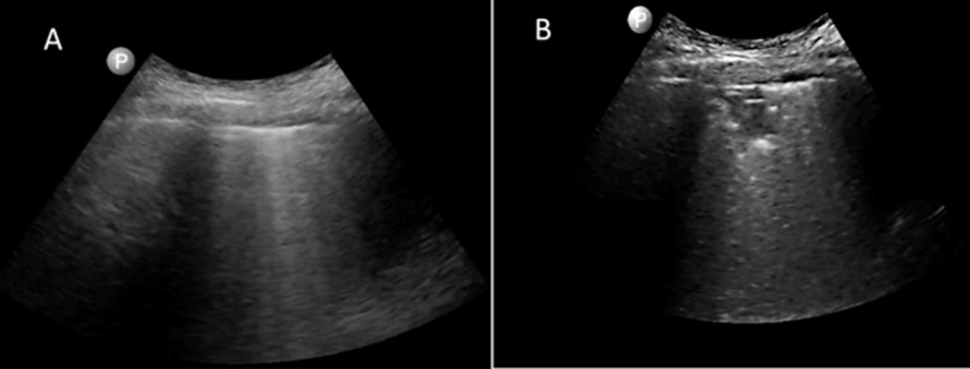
Lung ultrasound images taken using a curvilinear probe (C5 PhilipsLumify^TM^) in a patient admitted with COVID-19 pneumonia. Image of an interspace was saved in a six second loop. Both A and B are from the same interspace in the same loop. Image A seems to show B-lines originating from a smooth pleura and could easily be mistaken for pulmonary edema. Image B shows that during the respiratory cycle a subpleural consolidation with trace pleural effusion become evident. This case exemplifies why lung ultrasonography needs to be interpreted with caution when assessing volume status in patients with COVID-19.
